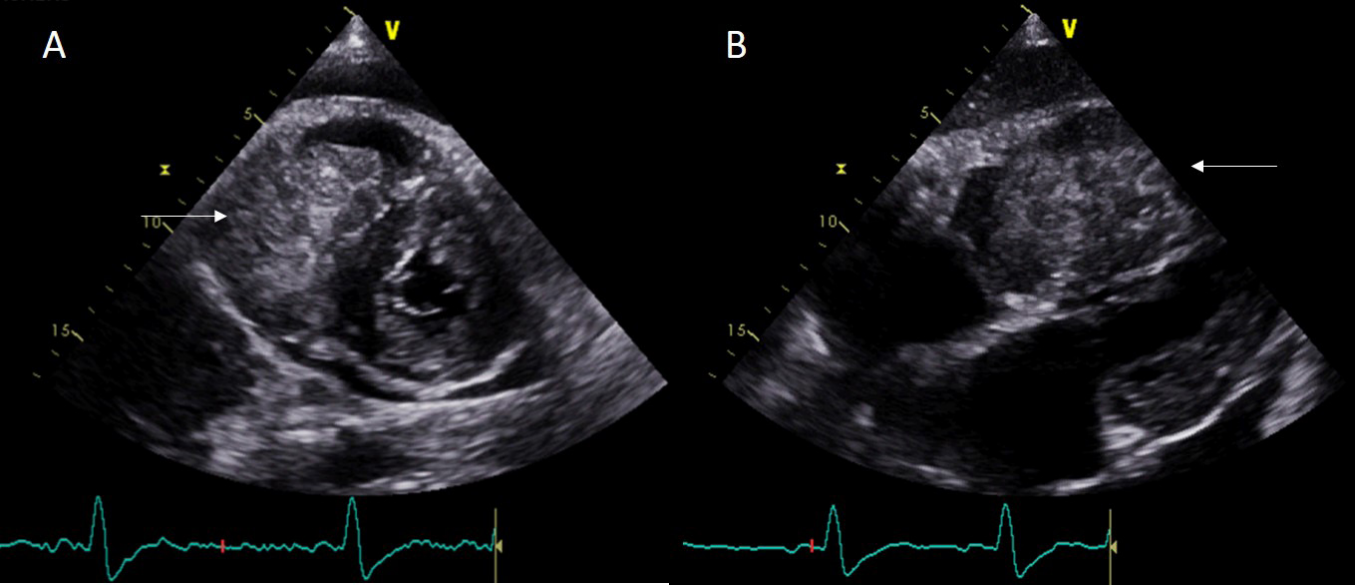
Transthoracic echocardiogram: Large mass in the right ventricle prolapsing
